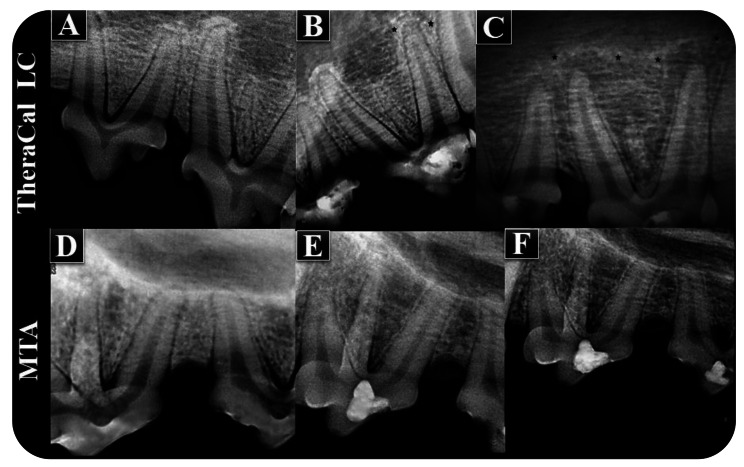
Periapical radiographic examination of teeth treated with TheraCal LC and MTA, respectively. (A and D) Preoperative periapical radiographs showing sound teeth and normal periapical tissues. (B and C) Postoperative periapical radiographs showing periapical pathology after 8 weeks of treatment with TheraCal LC before extraction. (E and F) Postoperative periapical radiographs showing no sign of periapical pathology after 8 weeks of treatment with MTA before extraction
